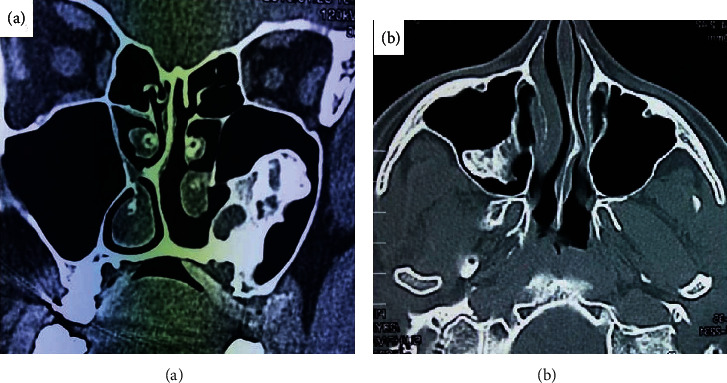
(a, b) CT scan showing a large mass in the left antrum, resting/supported on the floor of the antrum. No distinction of the medial antral wall was seen.
